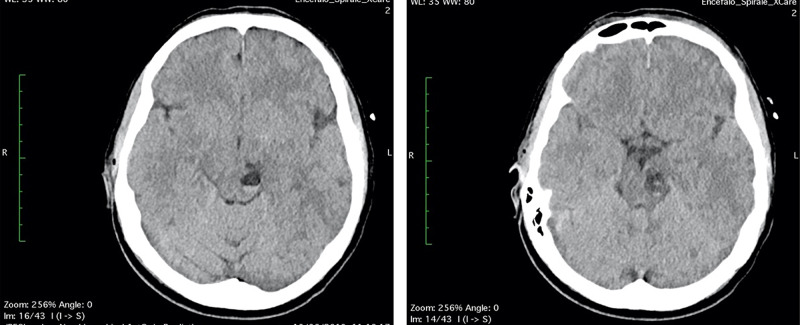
(A-B) Head CT scan “the day after”: the multiloculated cyst in the left midbrain showed fluid-fluid level, with enlargement of left cerebral peduncle and a slight mass effect, referring to enlargement and bleeding of PVSs. PVSs, perivascular spaces
